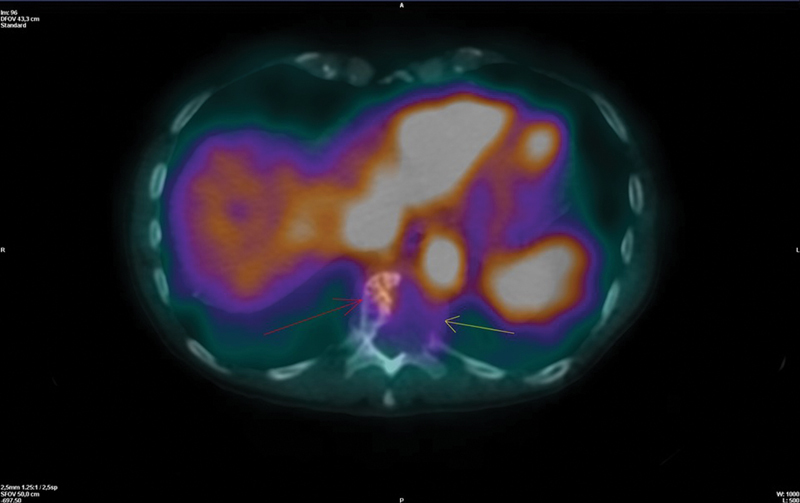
Axial slice of single-photon emission computed tomography/computed tomography fusion from the study of red blood cell scintigraphy showing the uptake of the radiotracer in the remaining bone of the T11 vertebra on the right (
